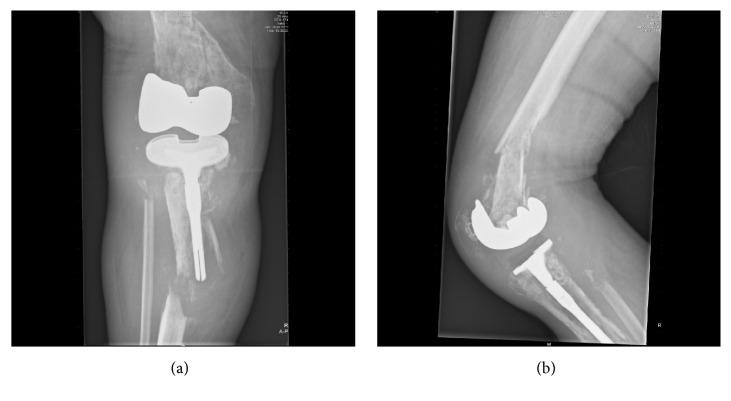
Case  3*. X-rays (anterior-posterior and sagittal) of failing total knee replacement, attributed to aggressive TGCT progression including bone involvement, after radiotherapy treatment.
